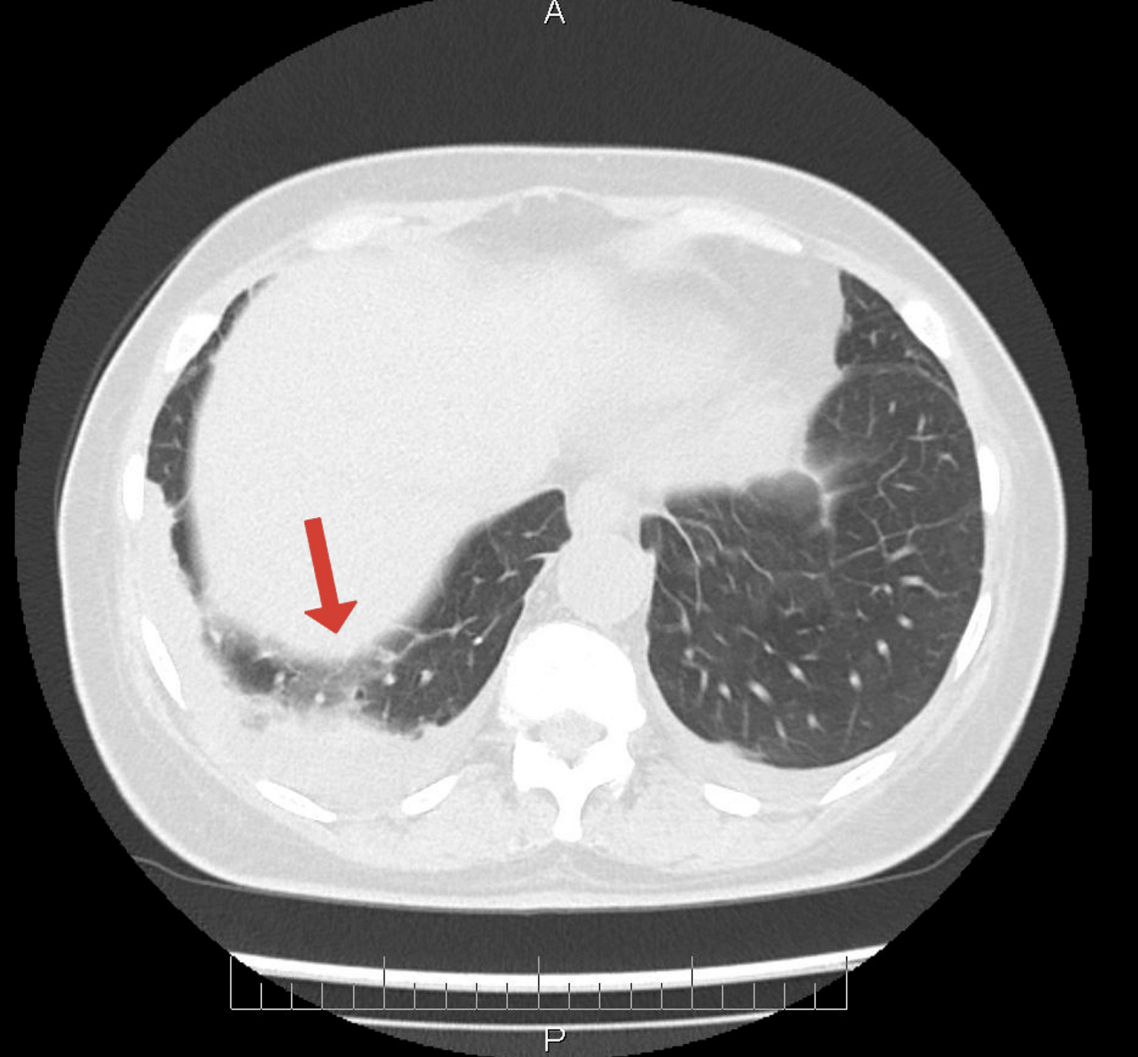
Non-contrast chest CT showing fibrotic changes and pneumonia. The arrow points out the most visible fibrotic changes and pleural effusion measuring 1.5 cm.
